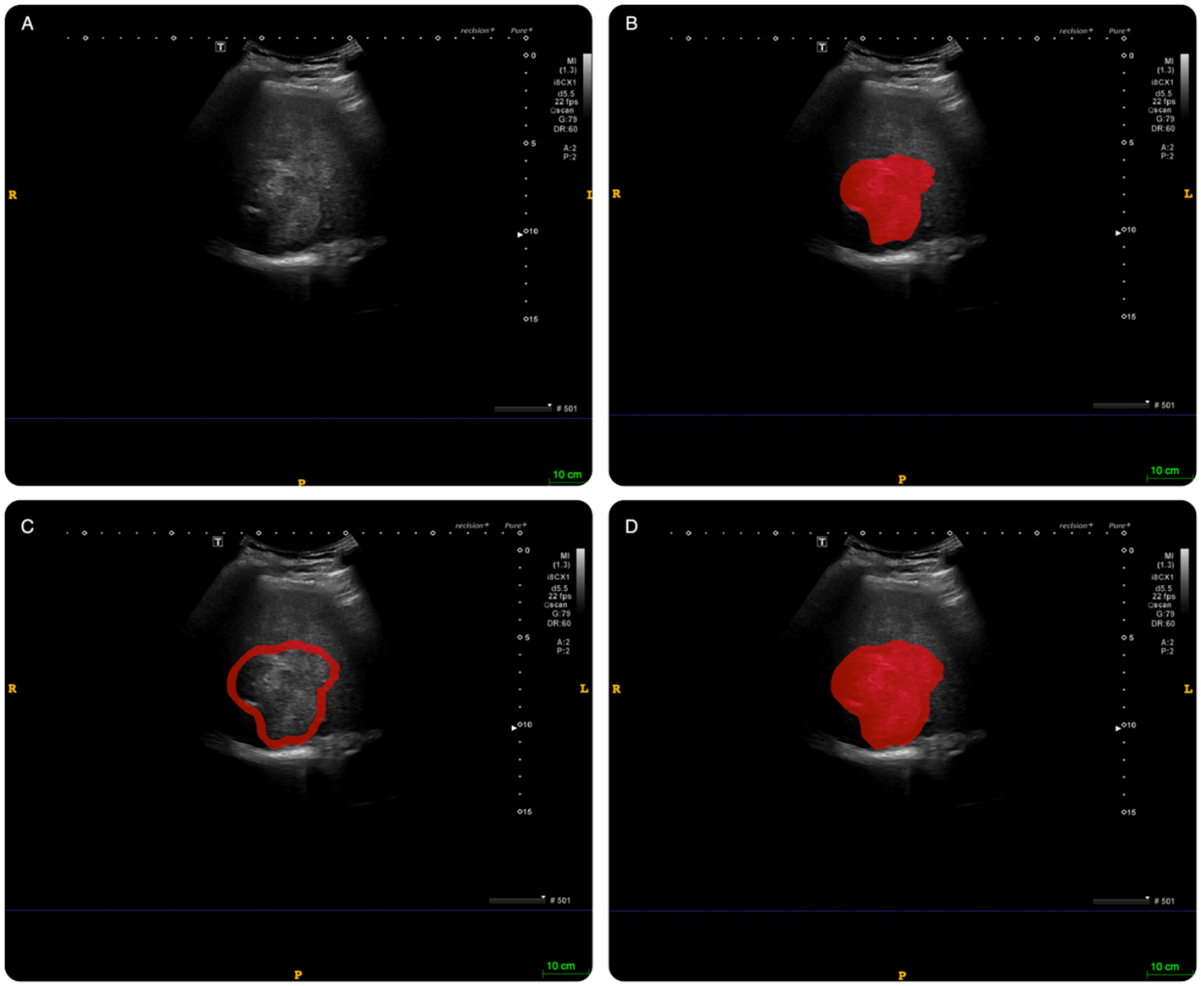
An example of delineating region of interest (ROI) on abdominal ultrasound imaging in ITK-SNAP software. (A): original image; (B): intratumoral ROI. (C): peritumoral ROI; (D): combined ROI (intratumoral tissue + peritumoral tissue).
